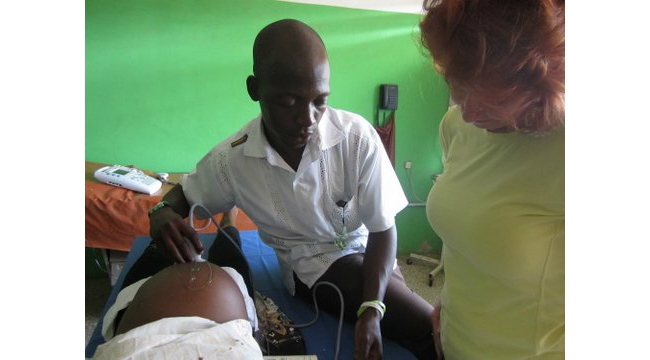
Hands-on ultrasound training of Ghanaian midwives by US-based registered diagnostic medical sonographer.
